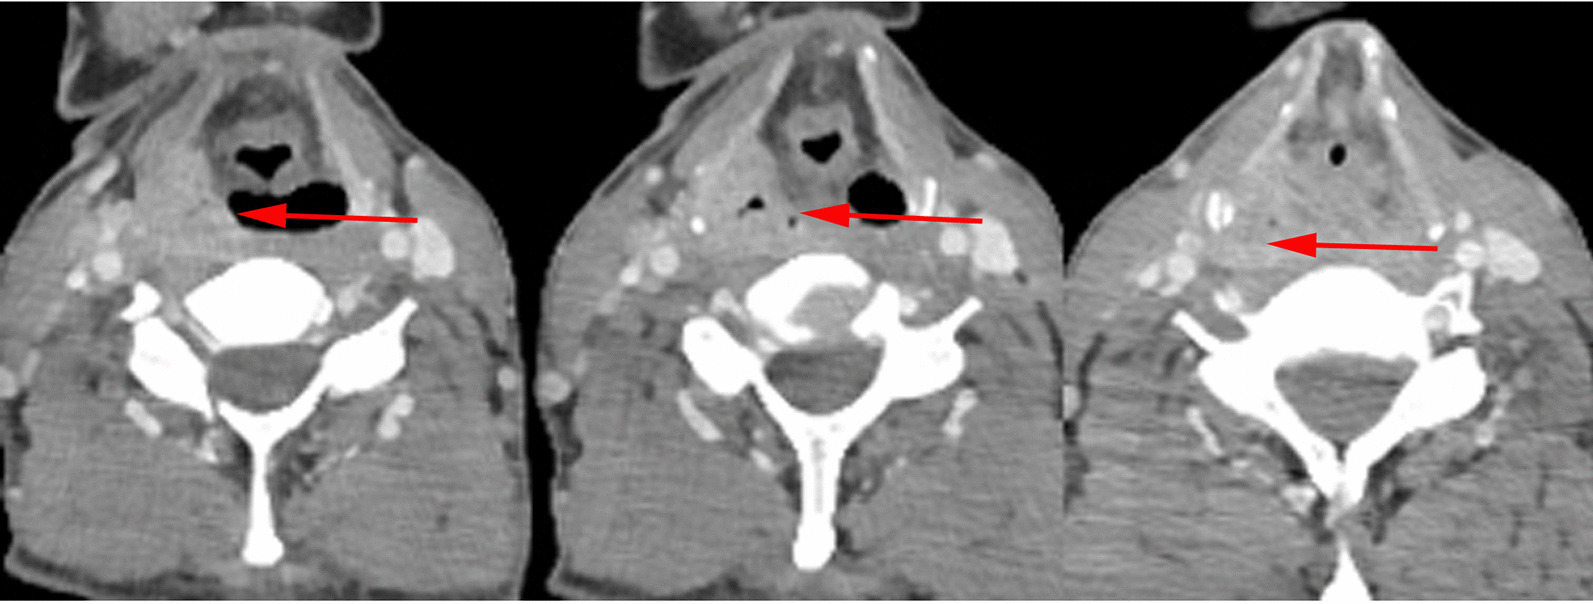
Preoperative contrast enhanced CT of one patient who was treated with the paraglottic approach. The arrow indicates the location of the tumor. The paraglottic space was identified not completely invaded and the esophagus was not involved
